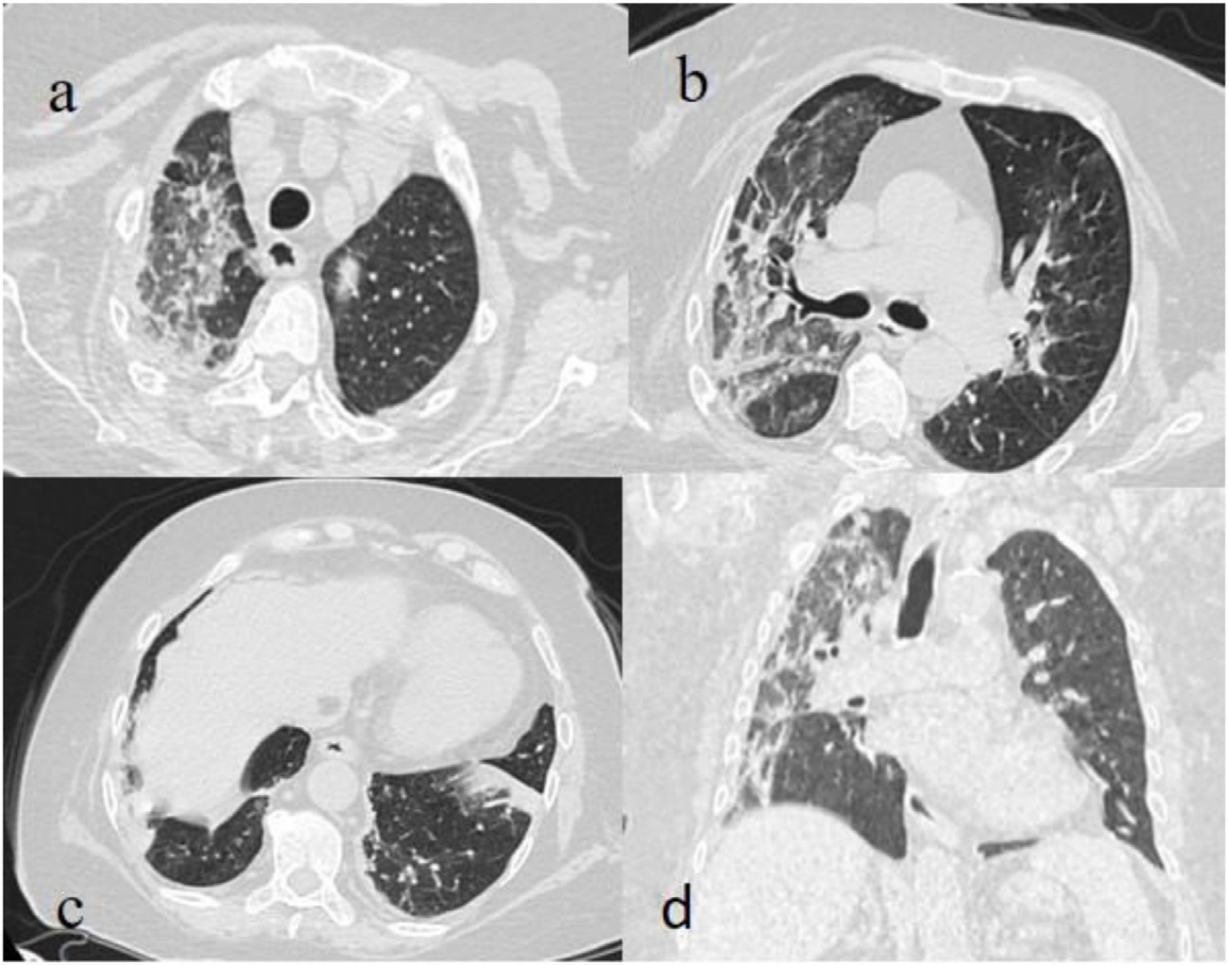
Chest CT performed at the baseline. In the images “a” and “b”, and “c” is represented the COVID-19 pneumonia with interstitial thickness with GGOs and initial consolidations in a multifocal distributions in the superior and inferior lobes with a predominance on the right side on the axial plane, in the image “d” on the coronal plane.
